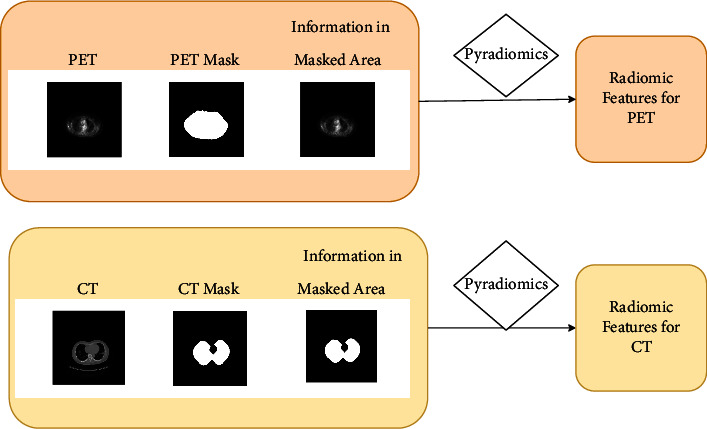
Image processing of CT and PET.
